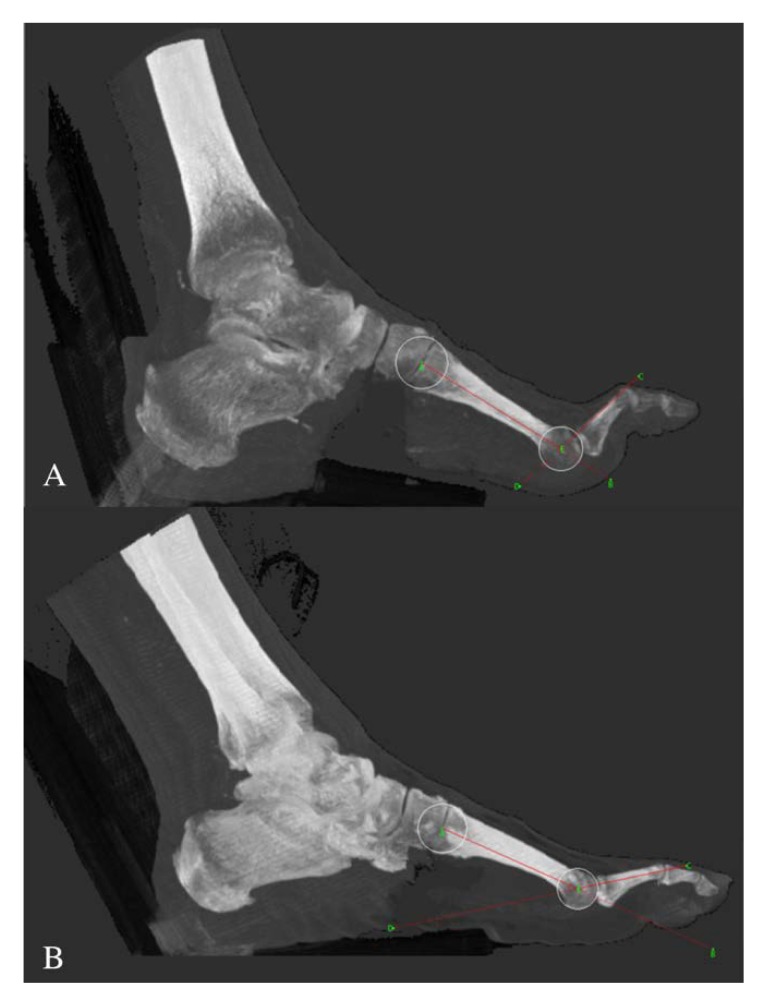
Representative CT scan images from individuals with severe (A, bone mineral density = 295 HU, 2nd metatarsophalangeal joint angle = 74.6 degrees) and minimal (B, bone mineral density = 489 HU, 2nd metatarsophalangeal joint angle = 36.1 degrees) metatarsophalangeal joint deformity. CT, computed tomography; HU, Hounsfield units.
